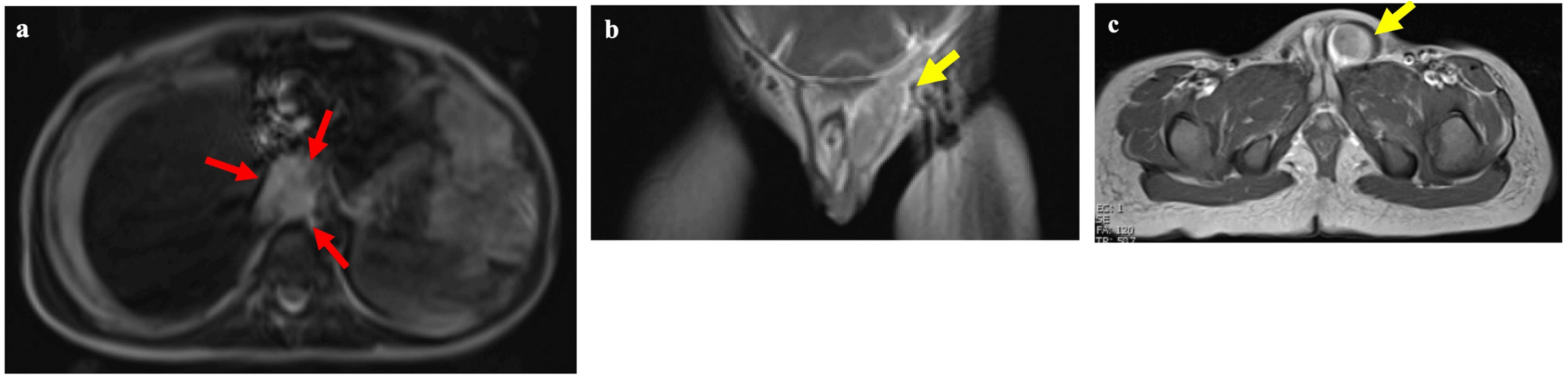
A contrast-enhanced magnetic resonance image of the chest and abdomen. The red arrows indicate the primary tumor lesion with mild hyperintensity on a horizontal T2-weighted image (a). The yellow arrow indicates a metastatic lesion extending from the left inguinal canal into the scrotum on a coronal contrast-enhanced T1-weighted image (b) and on a horizontal contrast-enhanced T1-weigted image (c). The size of tumors originating from the diaphragm in panel (a) was 18.19 x 24.26 mm, while that of tumors in the left inguinal region in panel (c) was 10.90 x 8.50 mm.
